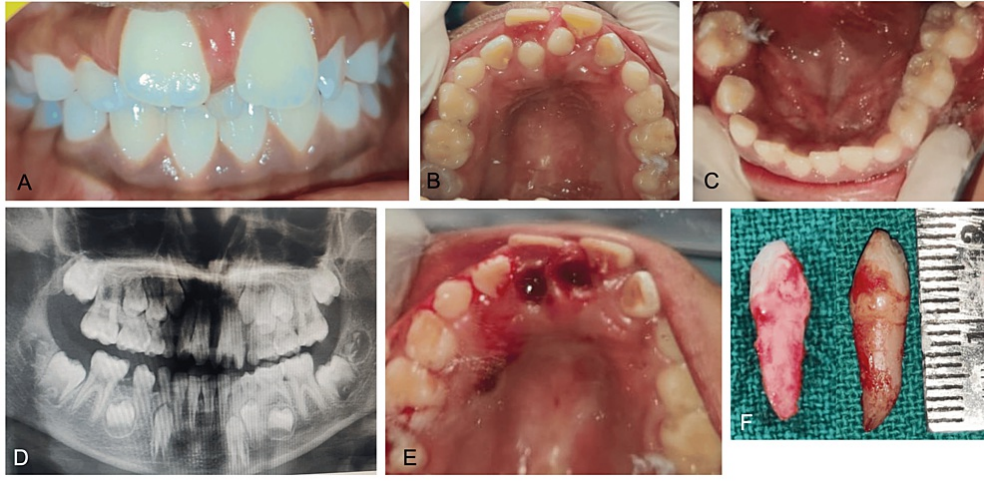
Case 3 A: pre-operative frontal view; B: pre-operative maxillary occlusal view; C: pre-operative mandibular occlusal view; D: pre-operative orthopantomogram (OPG); E: maxillary occlusal view; immediately post-extraction; F: extracted mesiodentes
